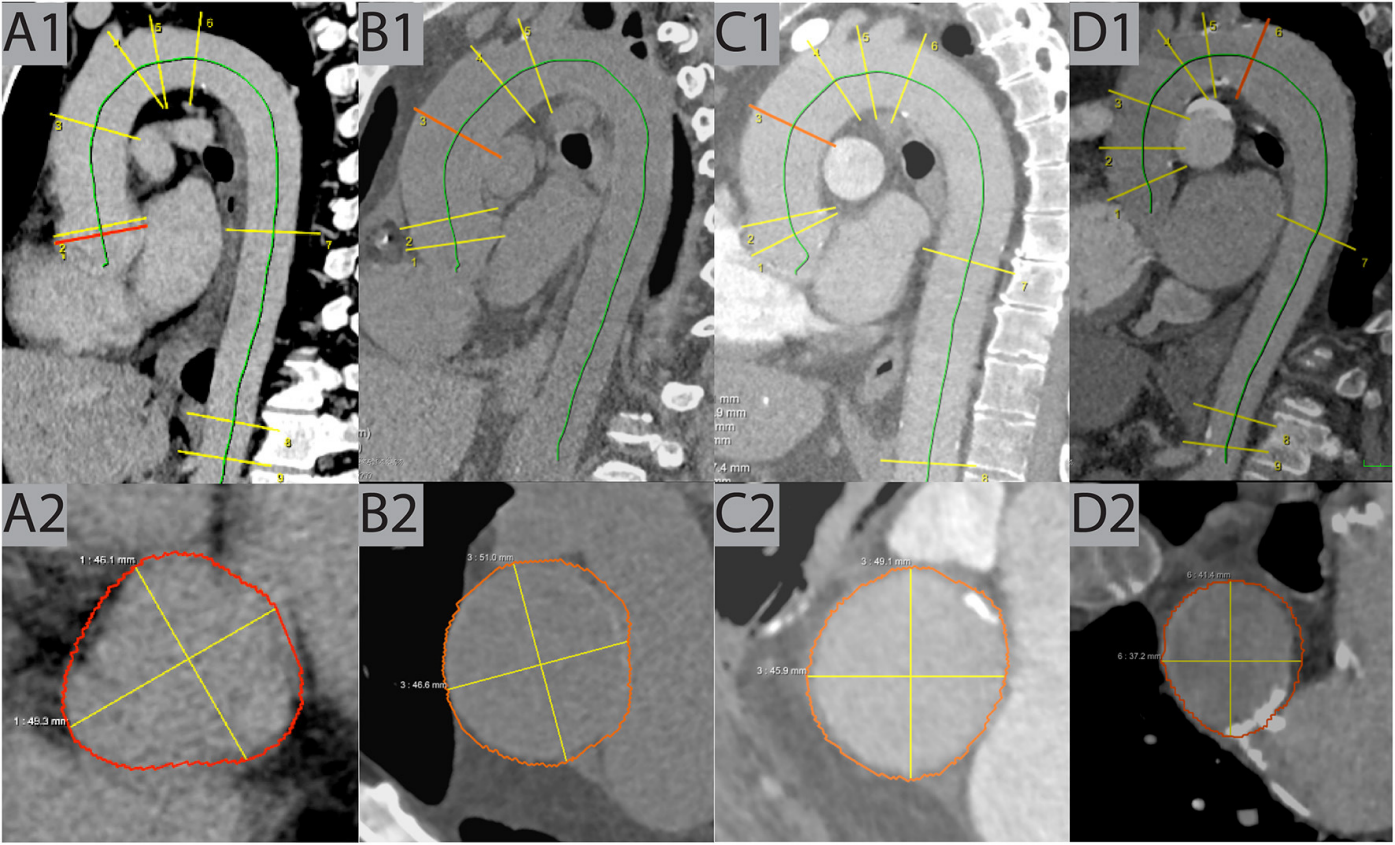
Examples of true positive cases. (A) This case shows dilatation of the AS (49 mm) in venous phase CT of a 57-year-old male patient detected by AIRad. (B) In this non-CE exam, dilatation of the AA (51 mm) was found in a 54-year-old male patient. (C) Similar to B but in pulmonary-arterial phase, AA dilatation (49 mm) in a 66-year-old male was revealed by AIRad. (D) Dilatation of the DA (41 mm) was identified by AIRad in a pulmonary-arterial phase CT of a 56-year-old female patient. AA, ascending aorta; AS, aortic sinus; CE, contrast enhanced; DA, distal arch.
